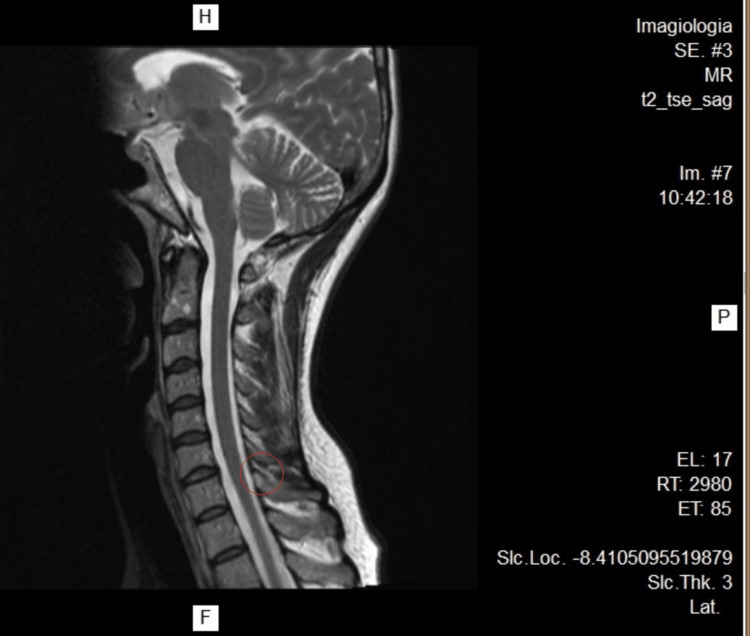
Cervical MRI. Without stenotic features
